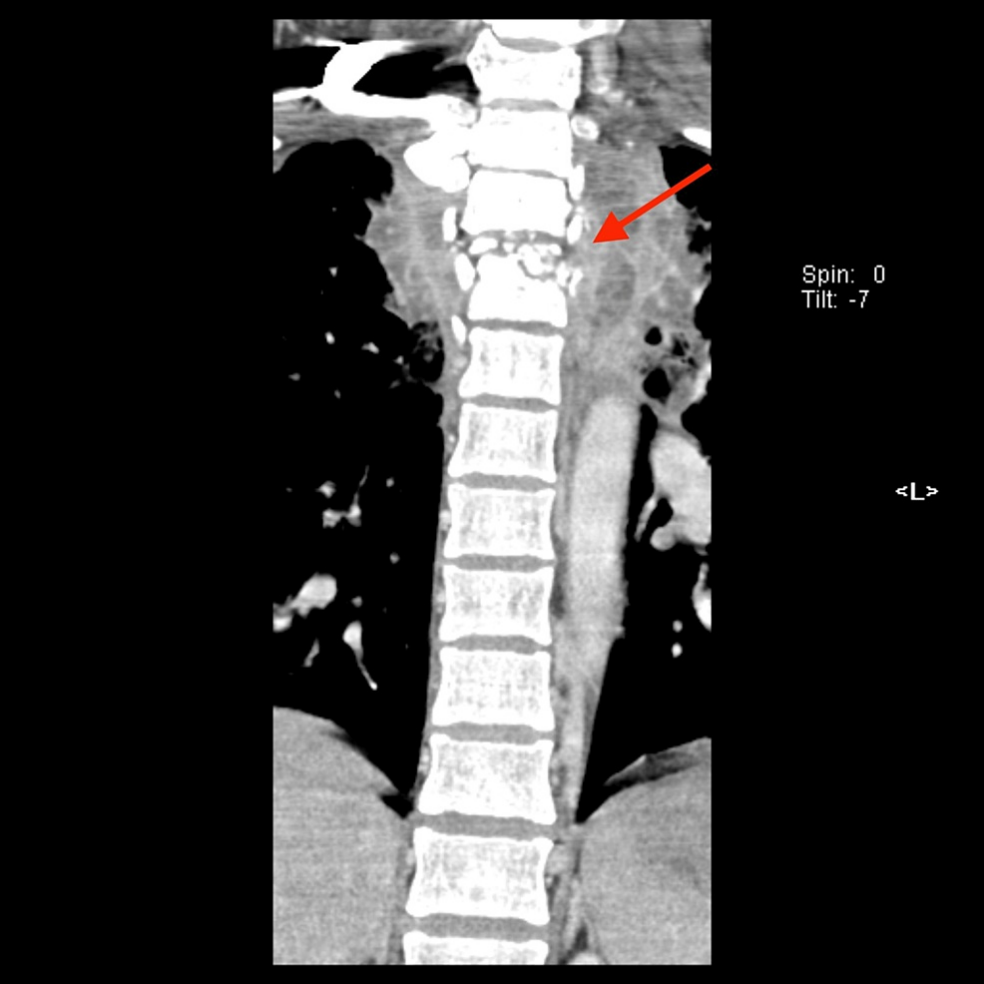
CT of the thoracic spine in a coronal plane Marked collapse and destruction of the T3 vertebral body.
